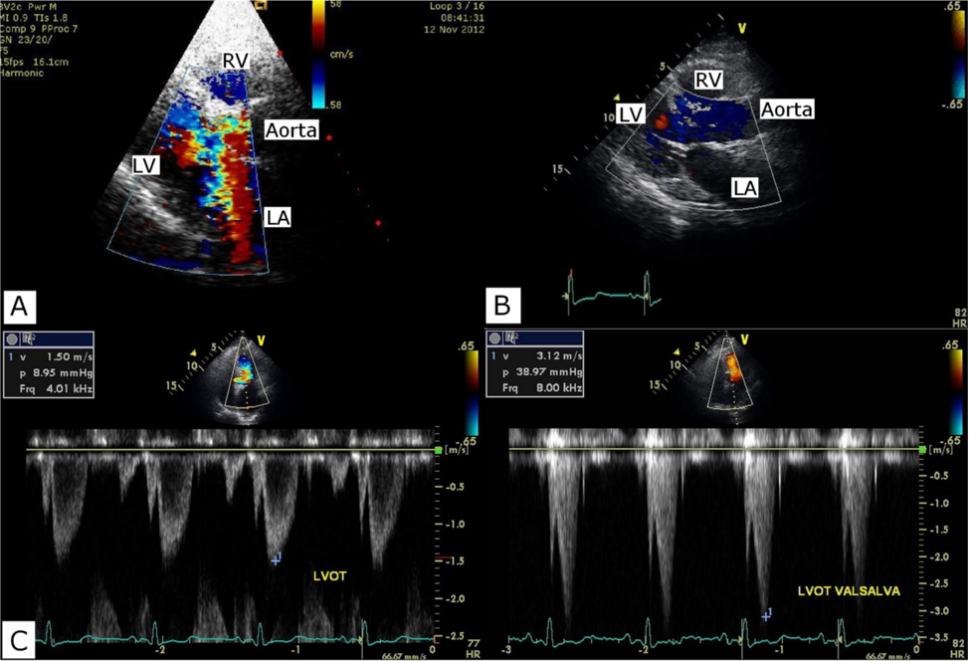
Echocardiography (A) Portable echocardiography that performed immediately after coronary angiography showed severe mitral regurgitation and systolic flow acceleration of left ventricular outflow tract obstruction on Hospital day 1. (B) On follow up echocardiogram after intra-aortic balloon pump weaning, mitral regurgitation completely disappeared. (Hospital day 3) (C) On follow up echocardiography after intra-aortic balloon pump weaning (Hospital Day 3), V_max_ is 1.5 m/sec and peak pressure gradient is 8.9 mmHg at resting state. At Valsalva maneuver, V_max_ is 3.1 m/sec and peak pressure gradient is 38.9 mmHg. LA, Left atrium; LV, Left ventricle; RV, Right ventricle; V_max_ , maximum velocity.
